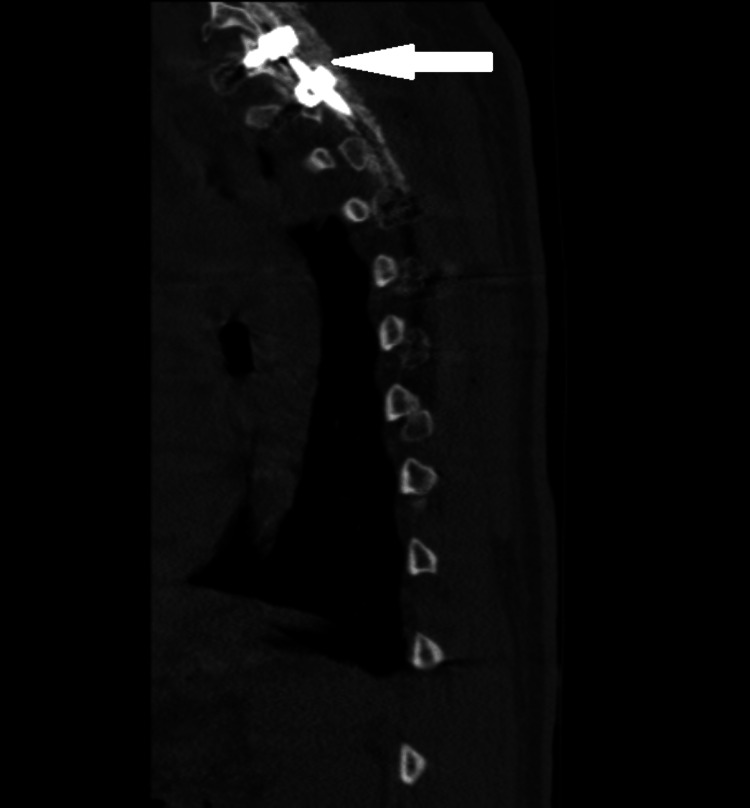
CT of the thoracic spine showing postoperative changes of T1 through T4 posterior instrumented fusion with multilevel laminectomy CT: computed tomography.
